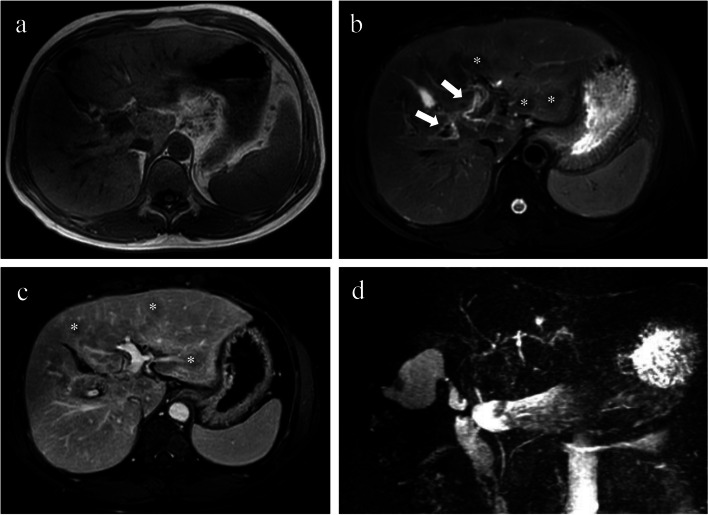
Contrast-enhanced MRI in a 53-year-old female with PBC (stage IV, Ludwig staging system) (a–d). The T1WI image shows the liver surface nodularity with an irregular contour (a). The T2WI image shows periportal hyperintensity (arrows) and periportal halo signs (*) (b). The PVP image shows the rounded low signal intensity lesions with no obvious enhancement (*) (c), and the MRCP image shows segmental irregular dilatation of the medium to small bile ducts (d)
